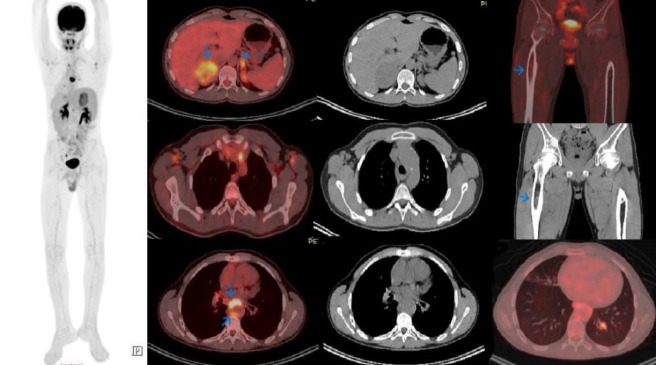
PET/CT Follow-up scan reveals FDG avid right adrenal mass (measuring 40×42mm, SUV_max_=5.2) and mildly FDG avid left adrenal nodule which are stable since previous PET study. Previously noted pulmonary nodule in RML resolved in current study and a new pulmonary nodule appears in the LLL (measuring 13mm, SUV_max_=4). Interval developed semi-circumferential intense FDG uptake (SUV_max_=20.5) is seen in the anterior descending thoracic aorta (10 to 1 o’clock below the carina), circumferential intermediate-grade uptake (SUV_max_=2.3) along the remaining thoracic aorta is also noted. Previously noted hypermetabolic axilla lymph nodes are again noted which are stable since previous study. Also, previously noted FDG-avid right femoral lesion now appeared as non-FDG-avid cortical thickening in the midshaft of femoral diaphysis, consistent with chronic or treated osteomyelitis
